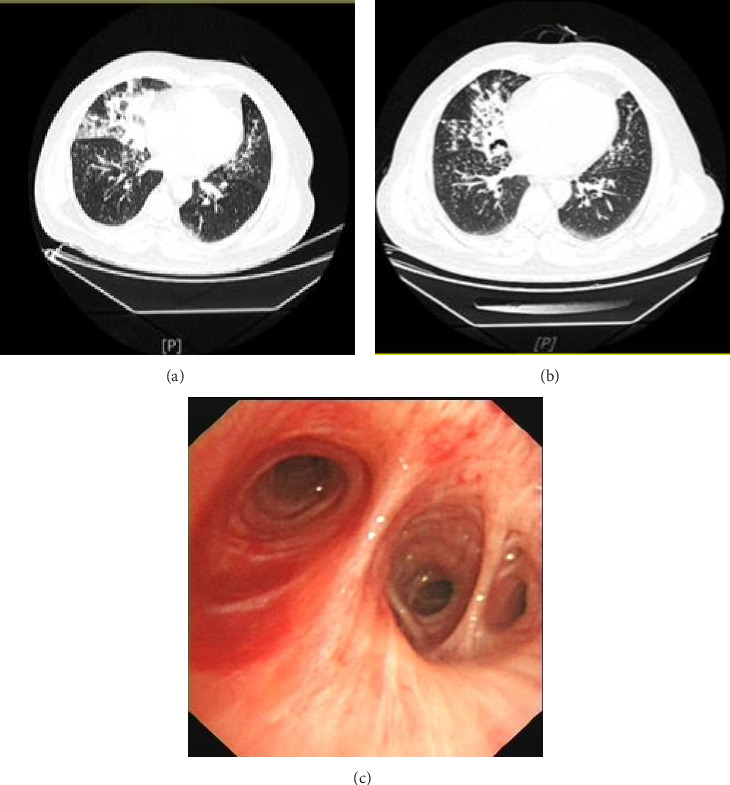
(a) August 18, 2022 chest CT showed multiple patchy solid shadows in both lungs. (b) August 26, 2022 chest CT showed a lesion in the middle lobe of the right lung that was significantly smaller than before. (c) August 18, 2022 bronchoscopy revealed bilateral bronchial mucosal congestion and edema, and a small amount of yellowish viscous secretion was visible in the lumen.
